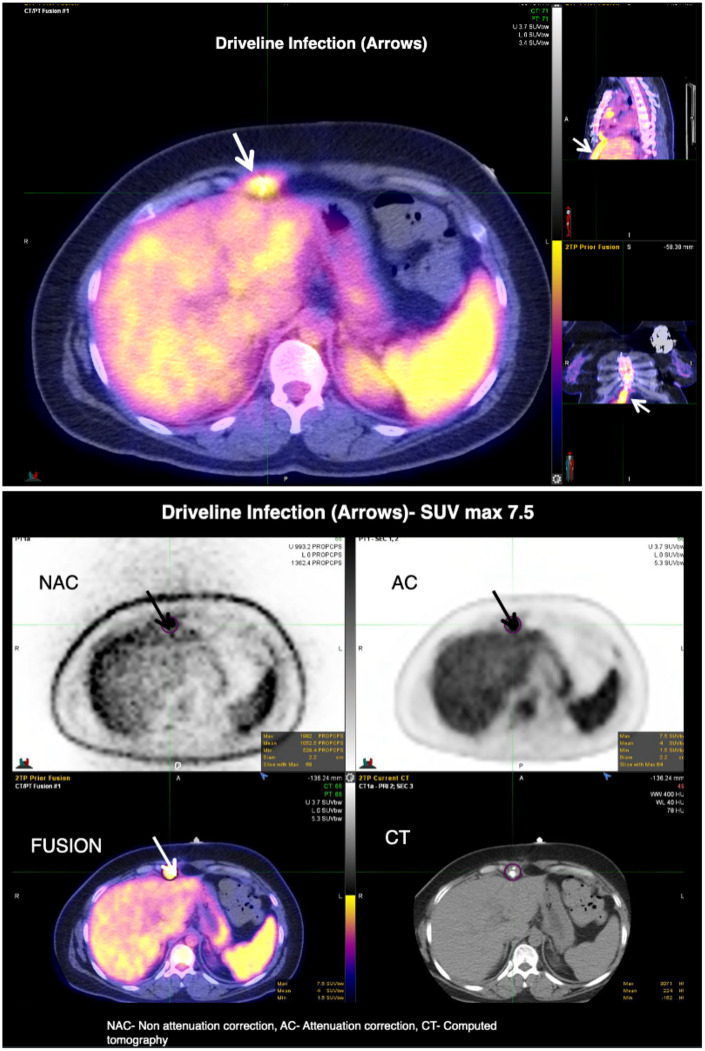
FDG-PET images of driveline infection.
